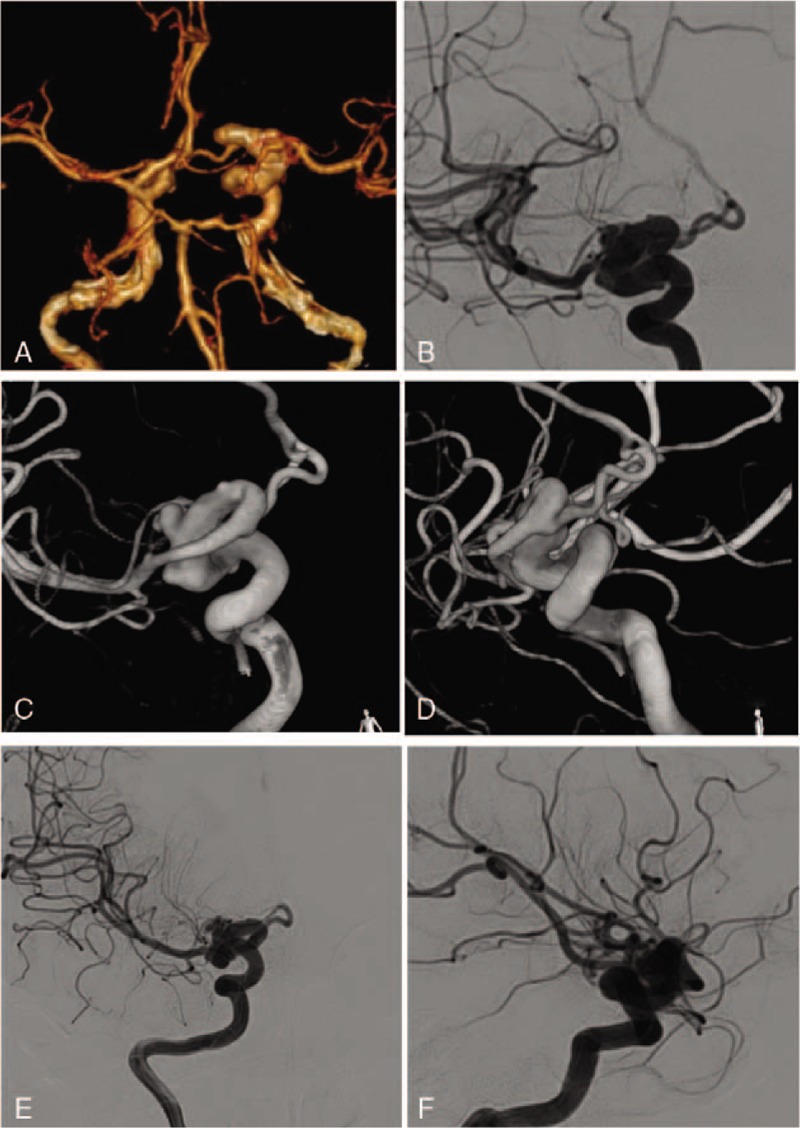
Images of the patient 2. A: Head CTA showing a ectatic and tortuous supraclinoid segment of the Right ICA; B: Right ICA injection angiograms showing a moderately ectatic and loosely coiled supraclinoid segment with 2 superimposed small aneurysms; C: Right ICA 3D injection angiograms; D: Right ICA 3D injection angiograms; EF: 6 mo FU.
